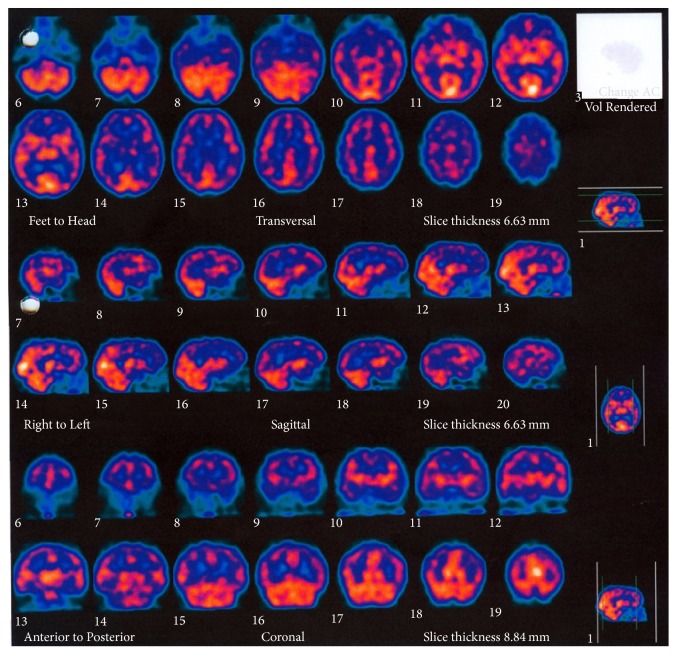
Brain perfusion SPECT. Reduced 99mTc-HMPAO uptake in parietal, temporal, and frontal lobes. Hypoperfusion is more severe on the left hemisphere.
